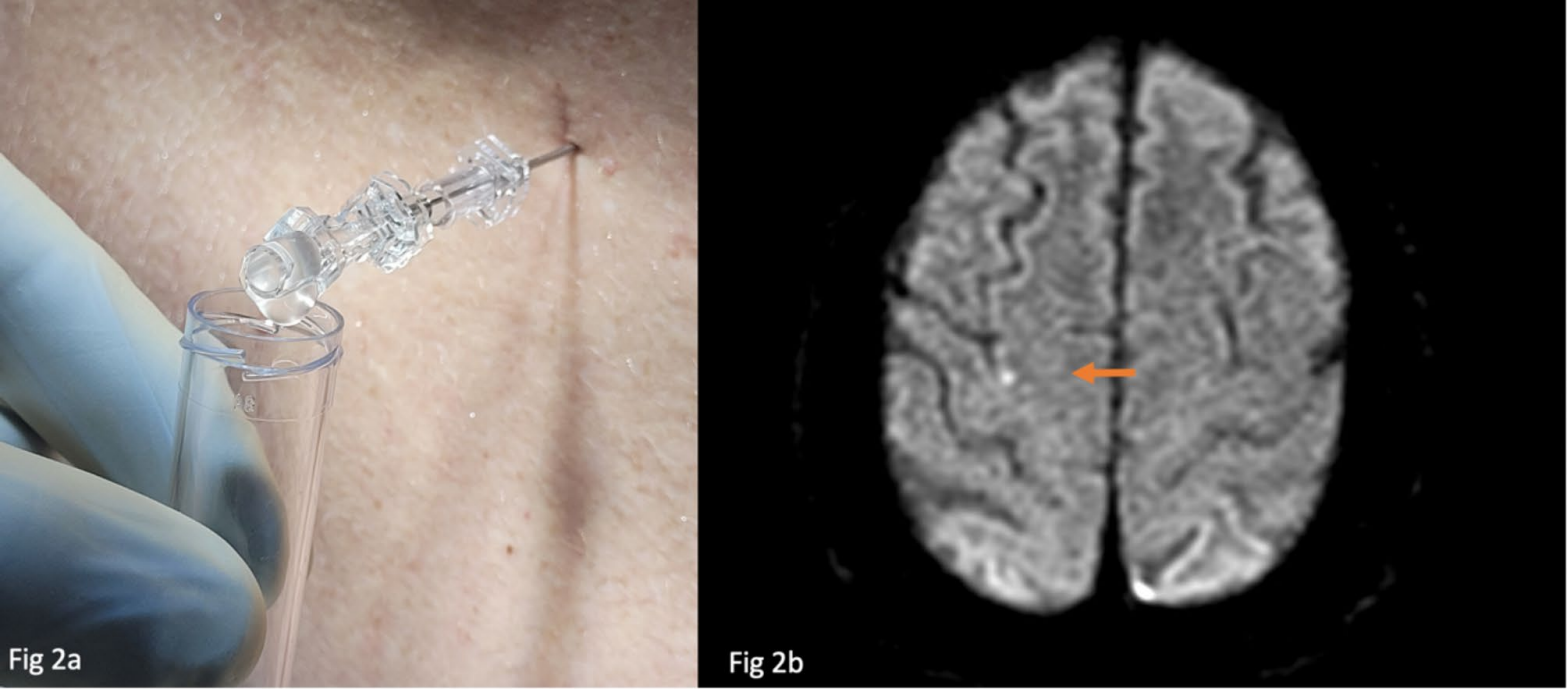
2a Macroscopic CSF fluid: turbid CSF detected at lumbar puncture indicating purulent meningitis. 2b: Axial MRI-DWI of the brain revealing diffusion restriction (arrow) indicating embolic stroke, highly suggestive of Austrian syndrome in the patient with pneumococcal meningitis and pneumonia
